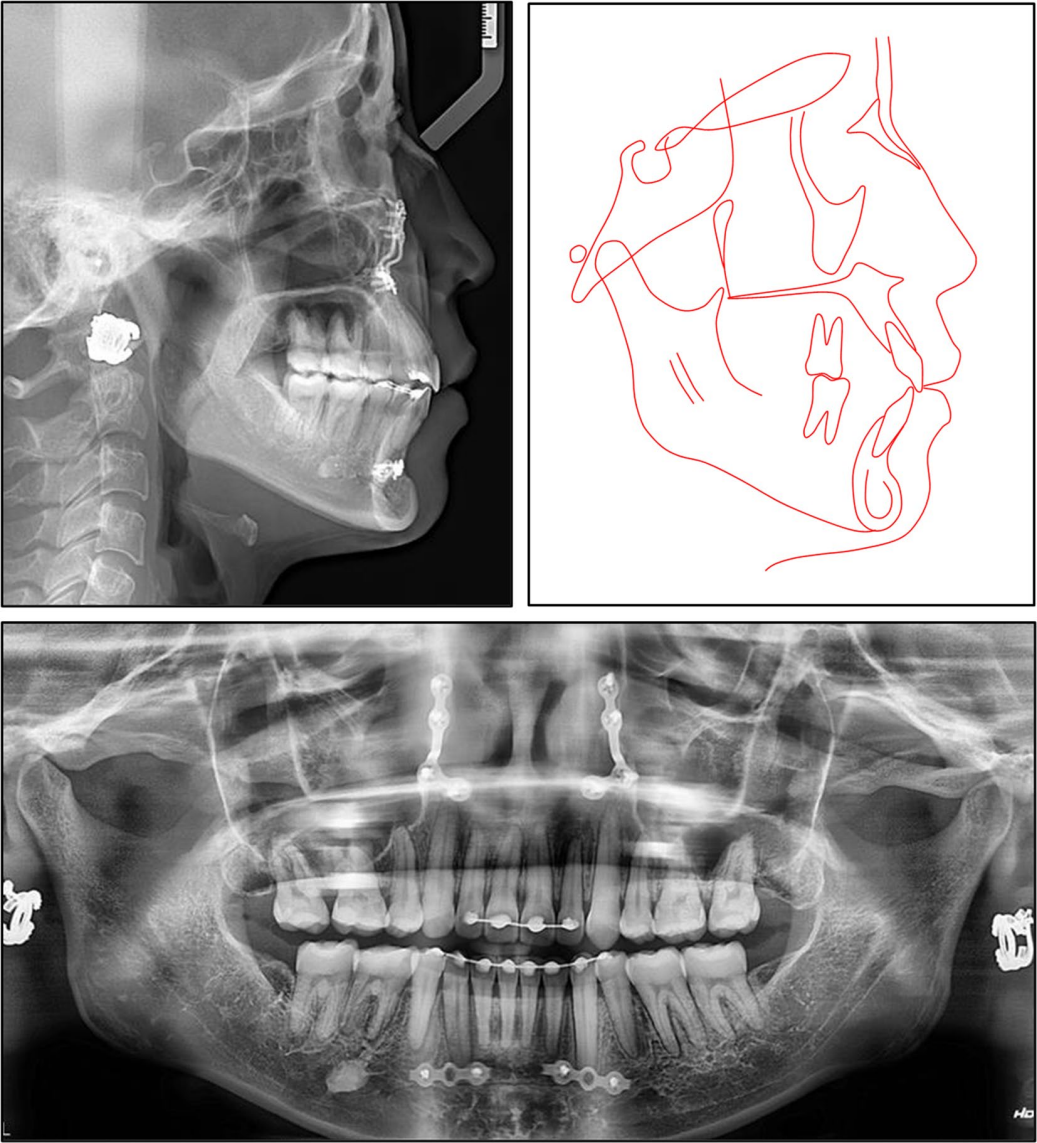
Post-treatment cephalometric and panoramic radiographs and tracing
